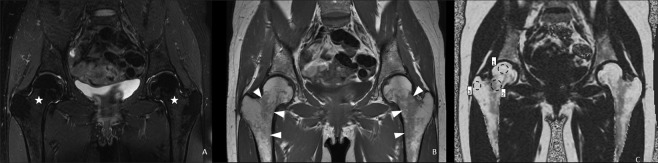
41-year-old female referred for the evaluation of chronic lower back and inguinal pain. (A,B) Coronal T2-weighted fat-saturated and T1-weighted images demonstrating a normal aspect of the proximal femora with a homogeneous low T2 signal (stars in A) and a high T1 signal with mottled areas of lower signal intensity corresponding to red marrow zones in the intertrochanteric region (arrowheads in B). (C) Proton density fat fraction map of the same patient demonstrating ROI positioning in the right femur. ROI 1, 2 and 3 are positioned in the femoral epiphysis (dashed circles), intertrochanteric region and greater trochanter respectively. Note that the ROI were placed strictly in the bone medullary cavity excluding cortical bone and peri-articular soft tissues.
